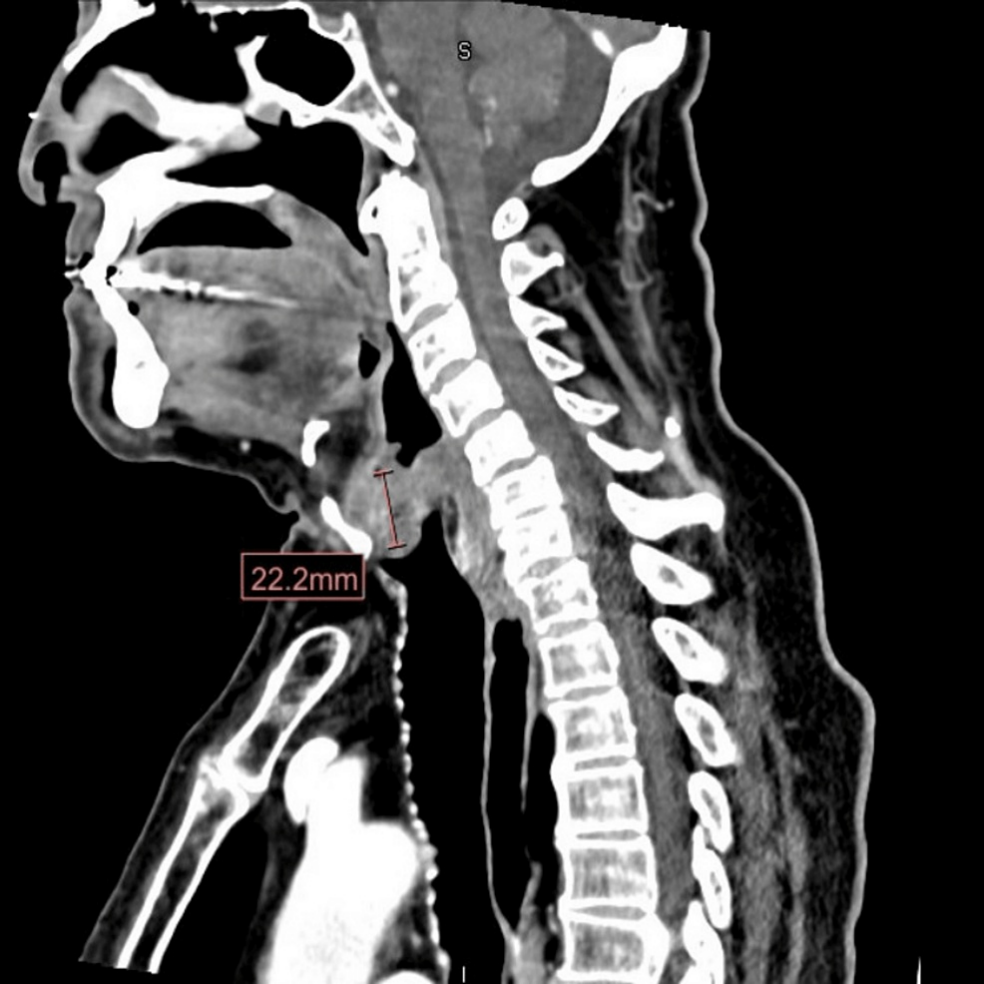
CT of the head and neck shows a lobulated mass centered along the anterior aspect of the larynx at the level of the vocal cords measuring approximately 2.2 × 1.4 cm CT: computed tomography
